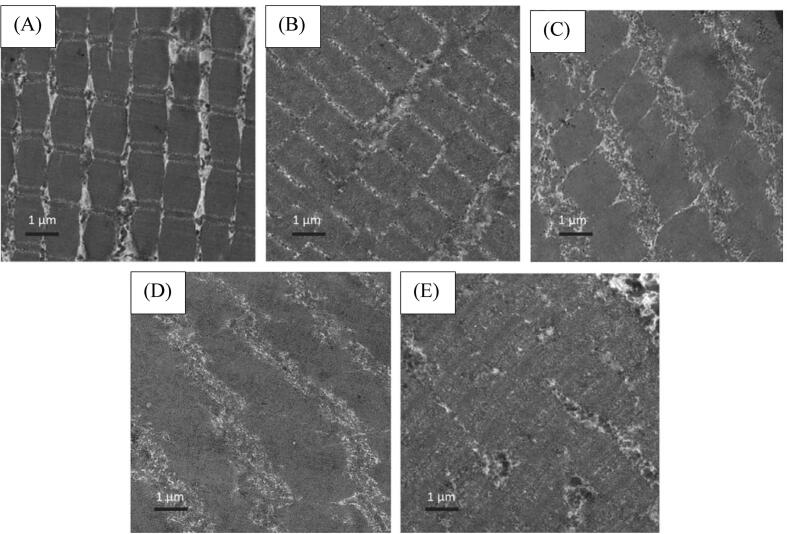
TEM images (3000 ×magnification, bar = 1 μm) of the center of the spiced beef with different ultrasound power with 120 min cooking time: (A) ultrasound power 0 W; (B) ultrasound power 400 W; (C) ultrasound power 600 W; (D) ultrasound power 800 W; (E) ultrasound power 1000 W [71].
